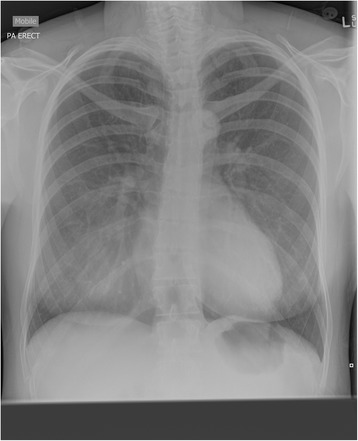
Chest X-ray demonstrating subcutaneous emphysema and pneumomediastinum
